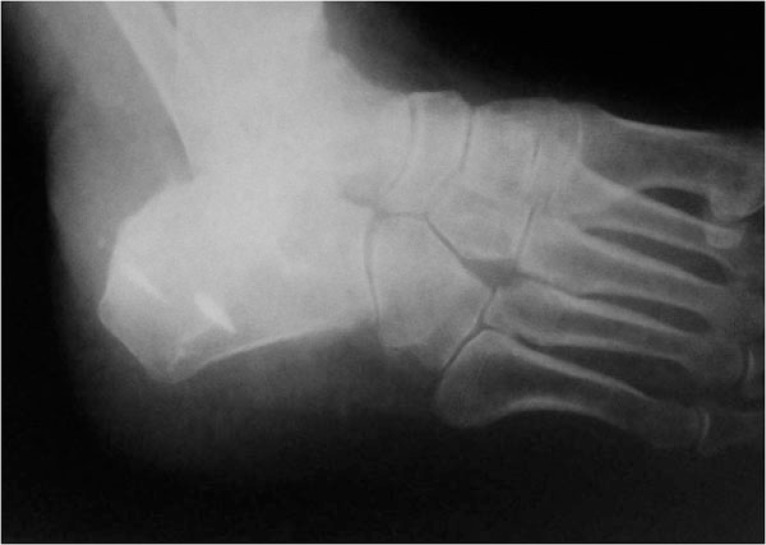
Lateral x-ray after reattachment of Achilles tendon with suture anchors.
